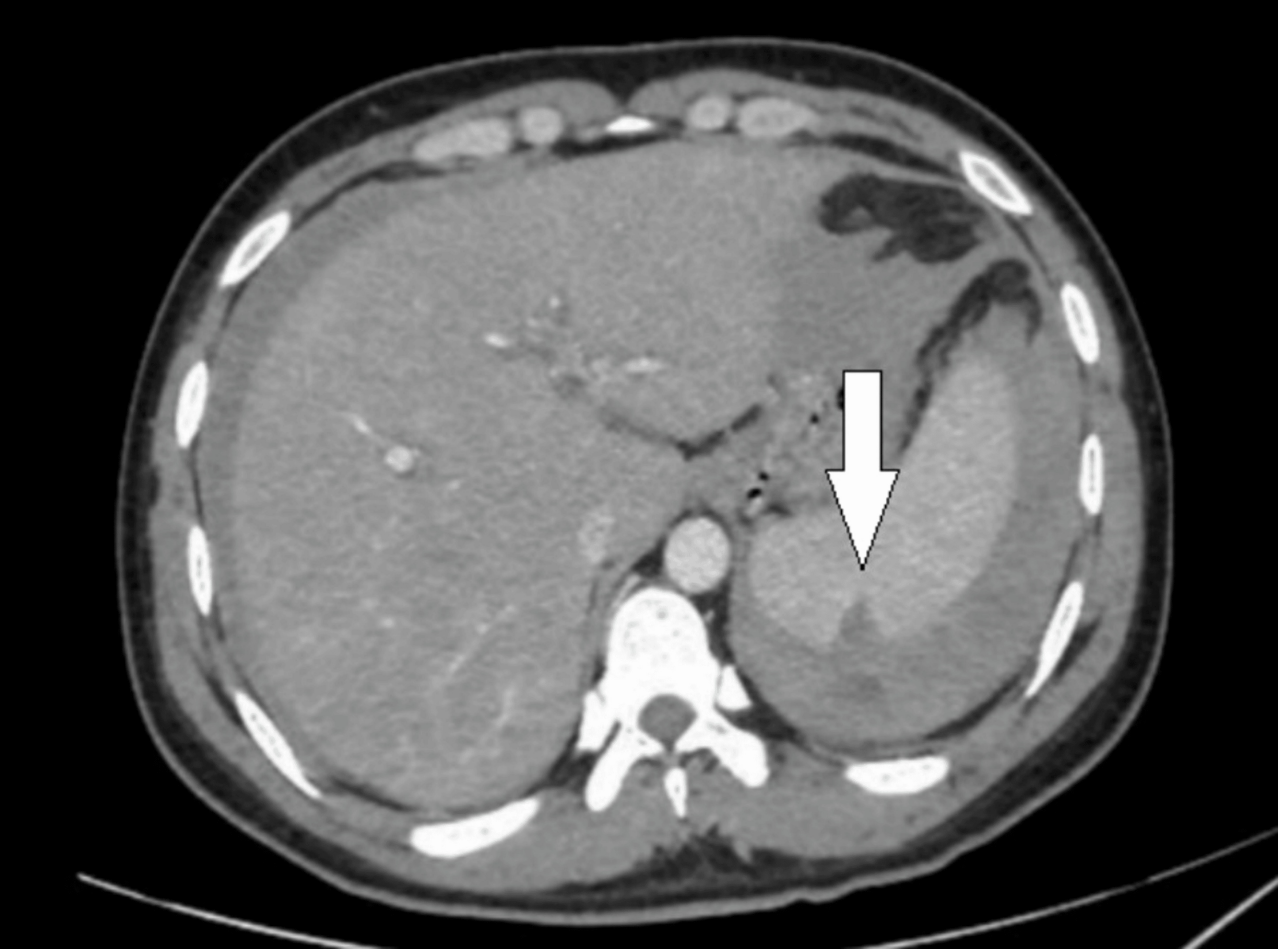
CECT abdomen on day 1 CECT: Contrast-enhanced computed tomography
